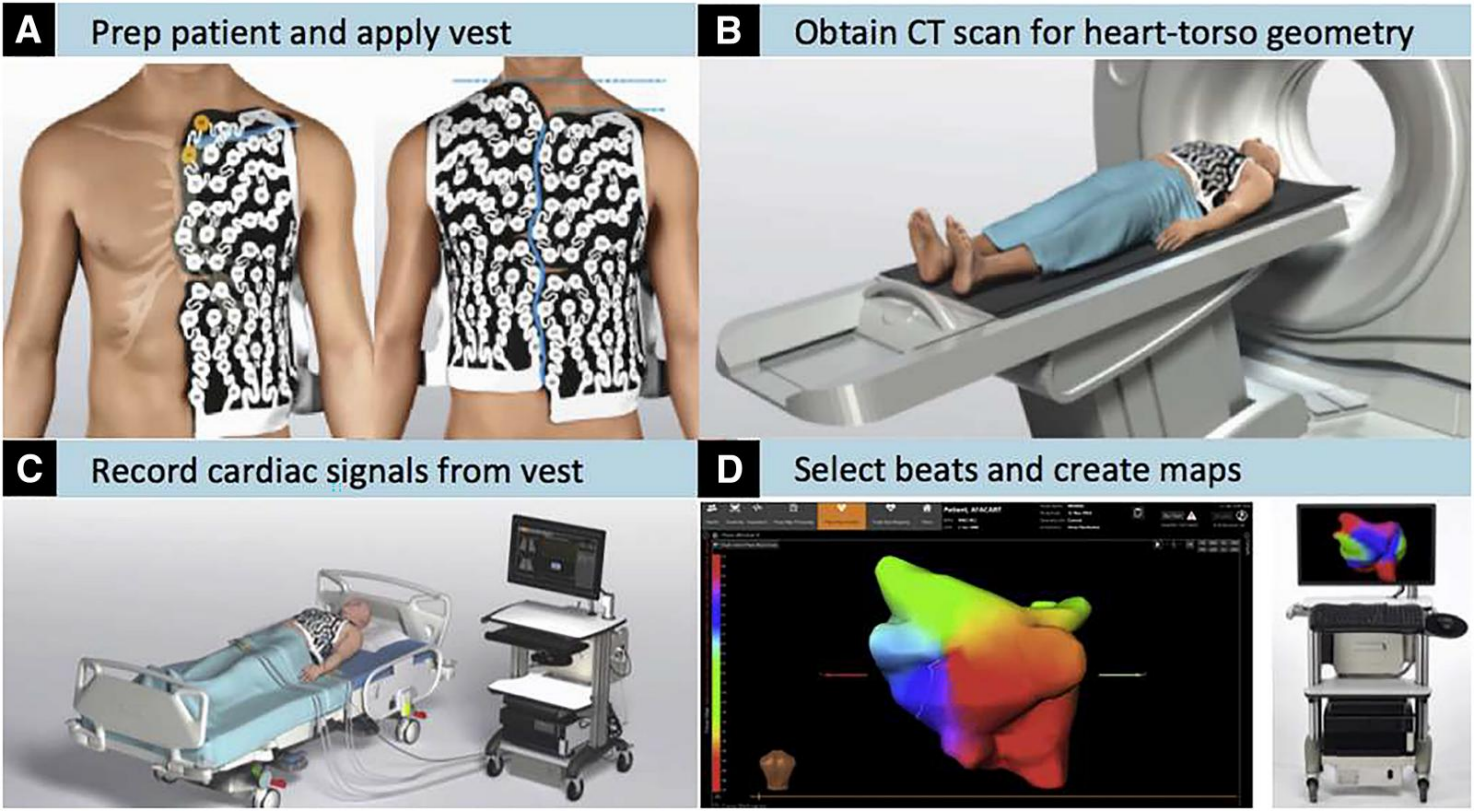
ECGI System. ECGI study: (A) The electrode vest is put onto shaved, dry skin, (B) CT scan, (C) recording, (D) 3D-reconstruction and reconstruction of the atrial surface potentials by the system’s algorithms. Figure reproduced with permission from the authors4 and Elsevier
